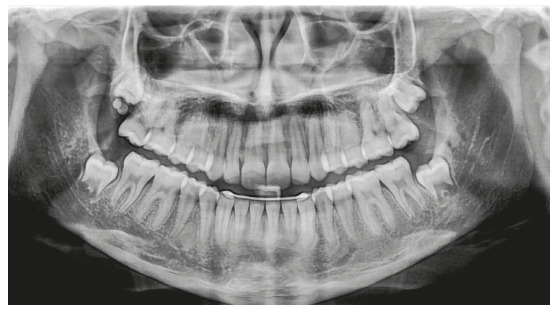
Case 1: Final panoramic radiograph.
